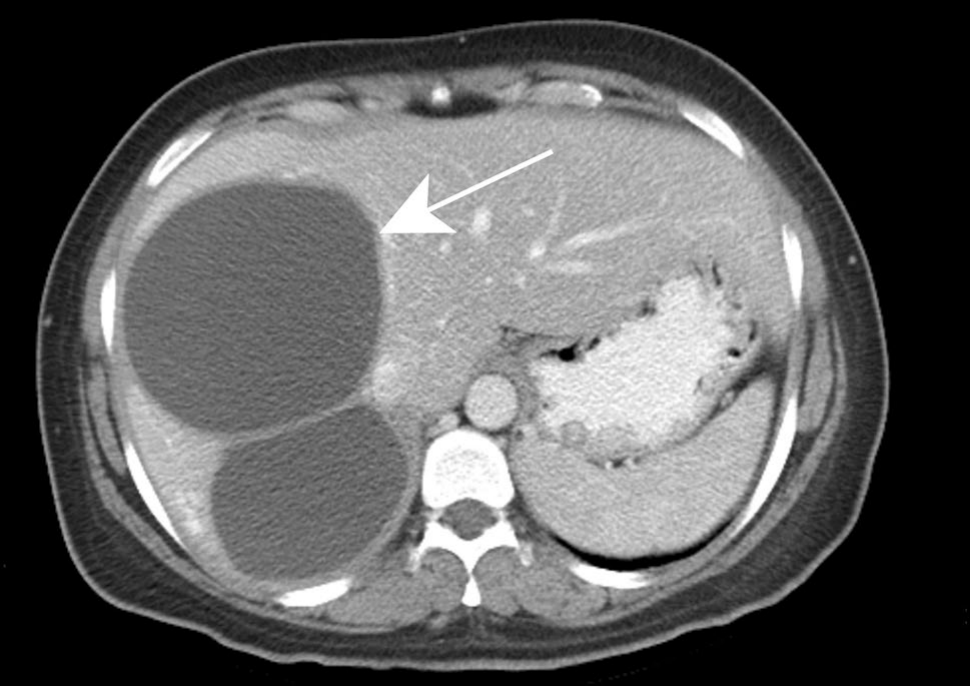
Cystic echinococcosis type CL in a 32-year-old female patient with upper abdominal discomfort. Axial contrast-enhanced CT image shows two large cystic lesions with thin walls in segment VII and VIII of the liver (white arrow). Ultrasound (not shown) revealed an anechoic cyst with a double layered wall, no internal daughter cyst or detached membrane
